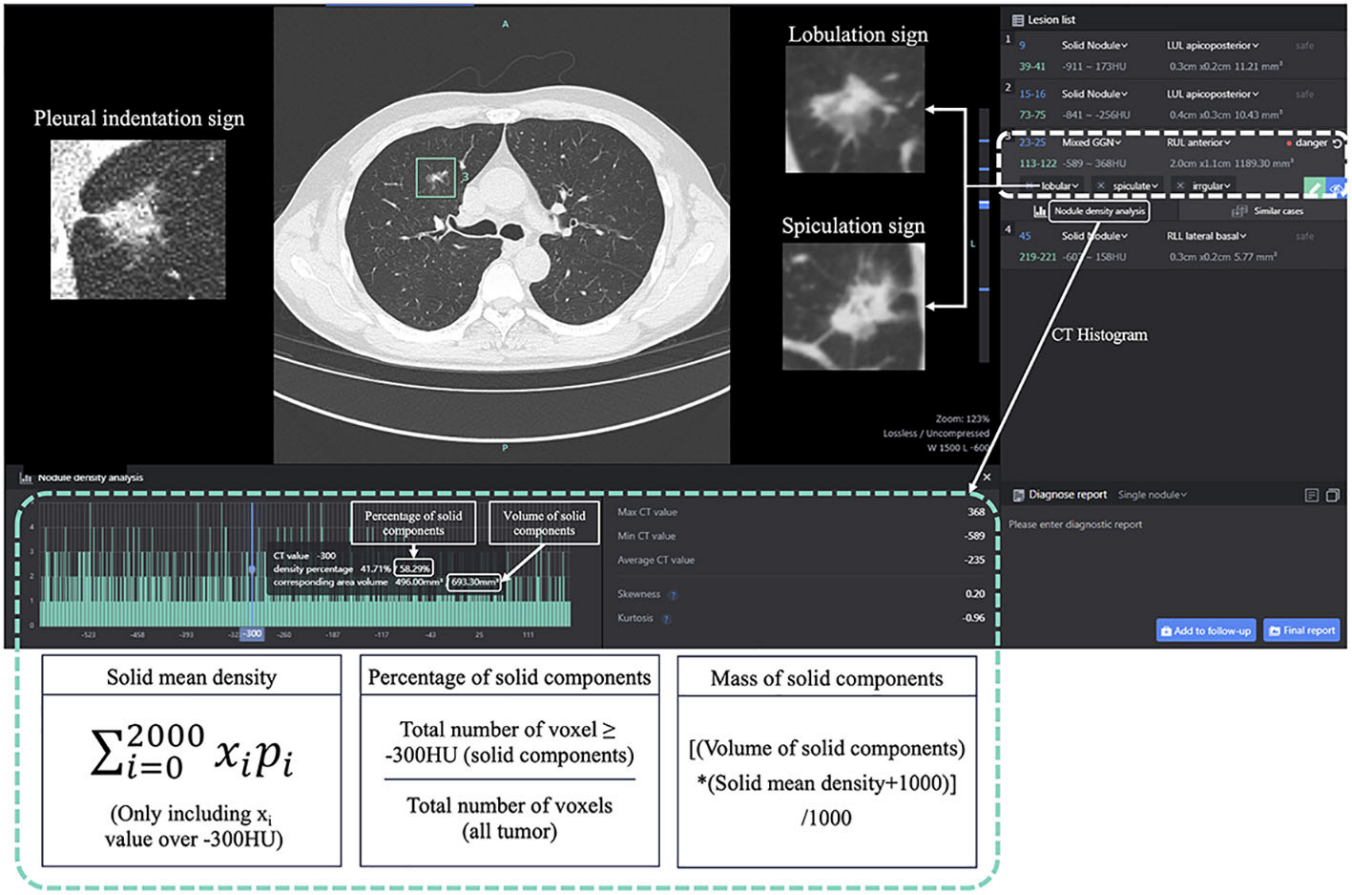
The CT histogram parameters using InferRead CT Lung, an AI-based pulmonary nodule auxiliary diagnosis system. Mixed GGN, mixed ground-glass nodule.
